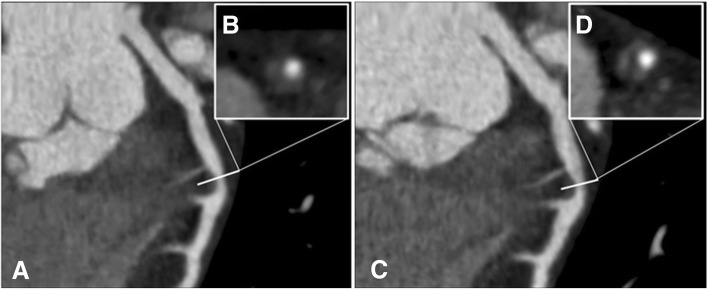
Example of non-calcified plaque progression at serial coronary computed tomography angiography. Automatically generated curved multiplanar reformation of image data of the left anterior descending coronary artery in an asymptomatic diabetic 46-year-old man performed in December 2010 (a) and in June 2013 (c). Displays of transverse sections perpendicular to the centerline show significant progression of the low-attenuation plaque volume from baseline (b) to follow-up (d) while the degree of coronary artery stenosis at this level worsens
